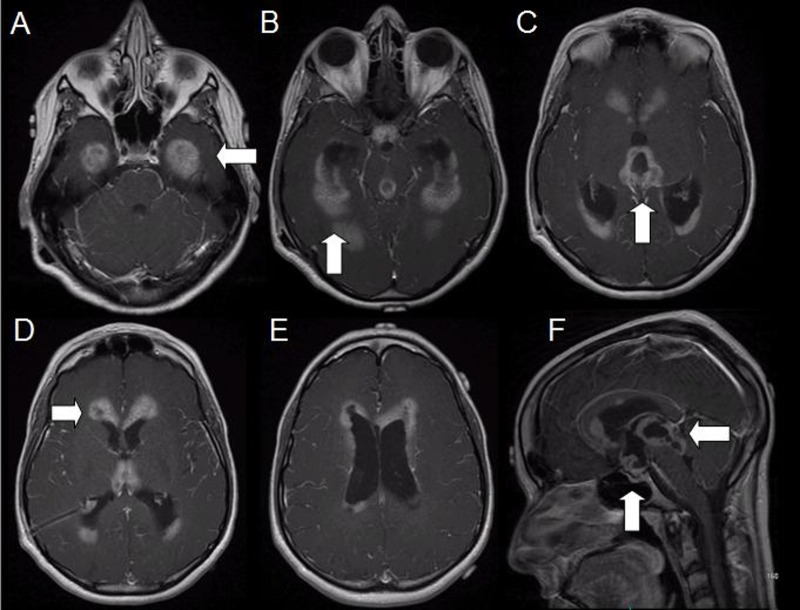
Post-contrast axial and sagittal MRI sequences with neuronavigation protocol at diagnosis. There is diffuse involvement of the lateral and third ventricles, the suprasellar and pineal regions, and the aqueduct with resultant ventriculomegaly.
